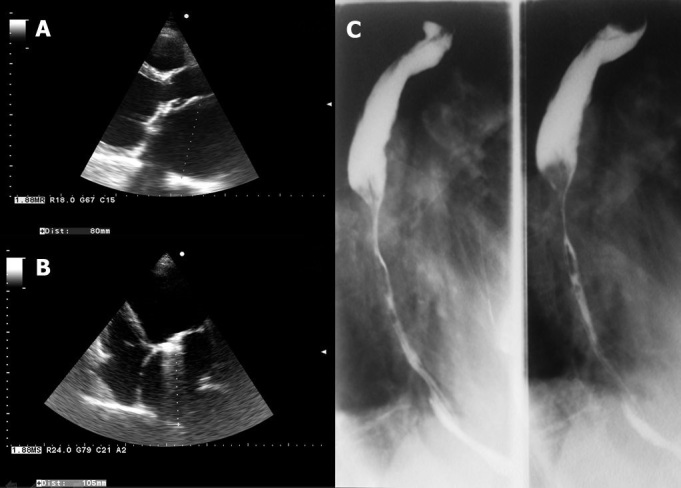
(A) echocardiography: parasternal long axis view, left atrial postero-anterior diameter = 80mm; (B) echocardiography: apical four chamber view, left atrial longitudinal diameter = 105mm); (C) X-ray examination with barium of the upper gastrointestinal tract demonstrating significant lumen reduction in the middle and lower part of the esophagus
